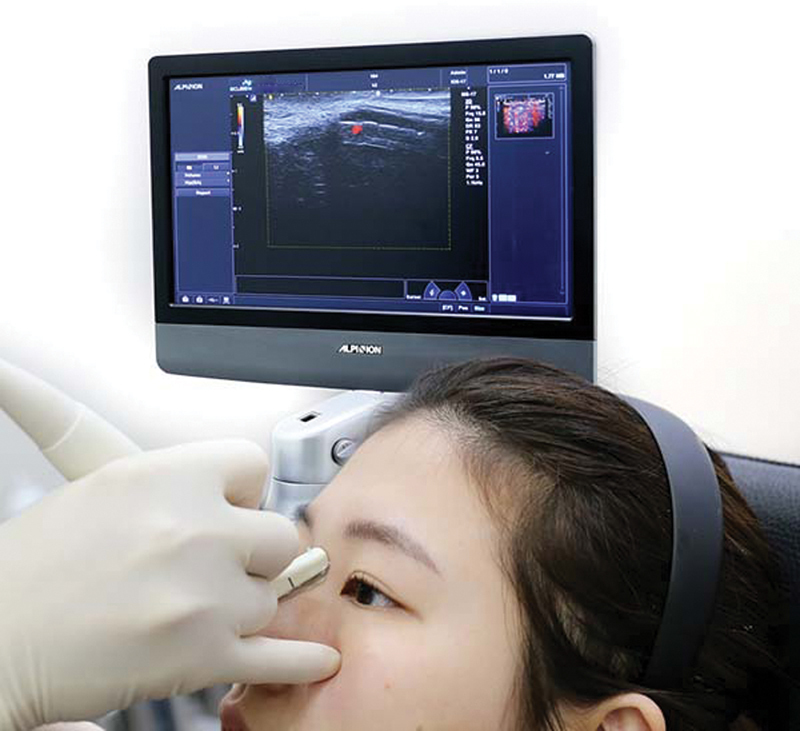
Doppler ultrasound detection at nose.
